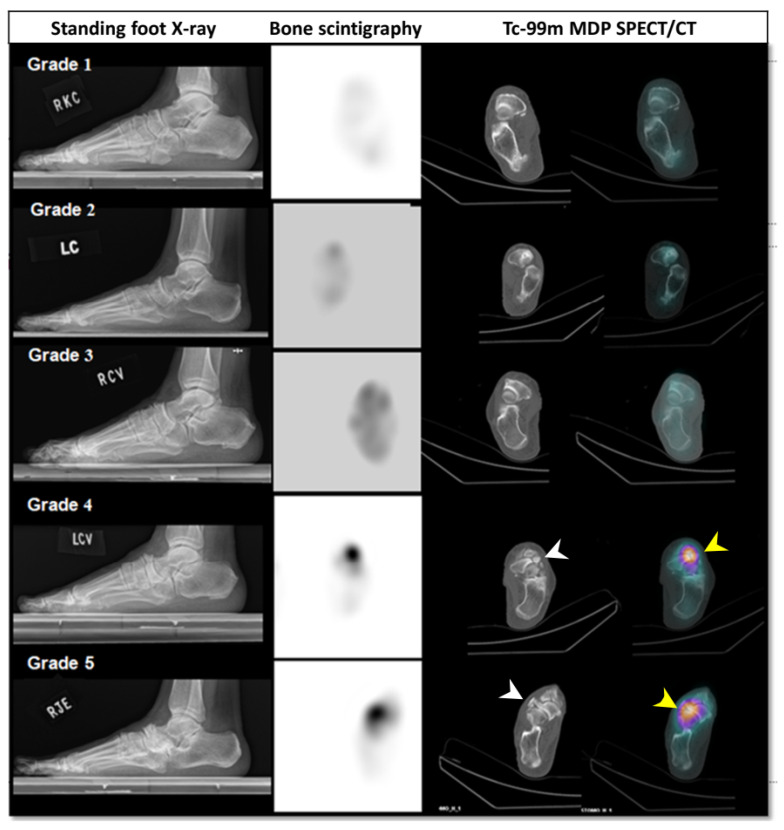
The associated changes in the images of bone scintigraphy and SPECT/CT in MWS cases of different Maceira stages. The white arrowheads in SPECT/CT panels indicate the navicular fragmentation and deformity. The significant higher radiotracer uptake at talonavicular joint in SPECT/CT panels (yellow arrowheads).
